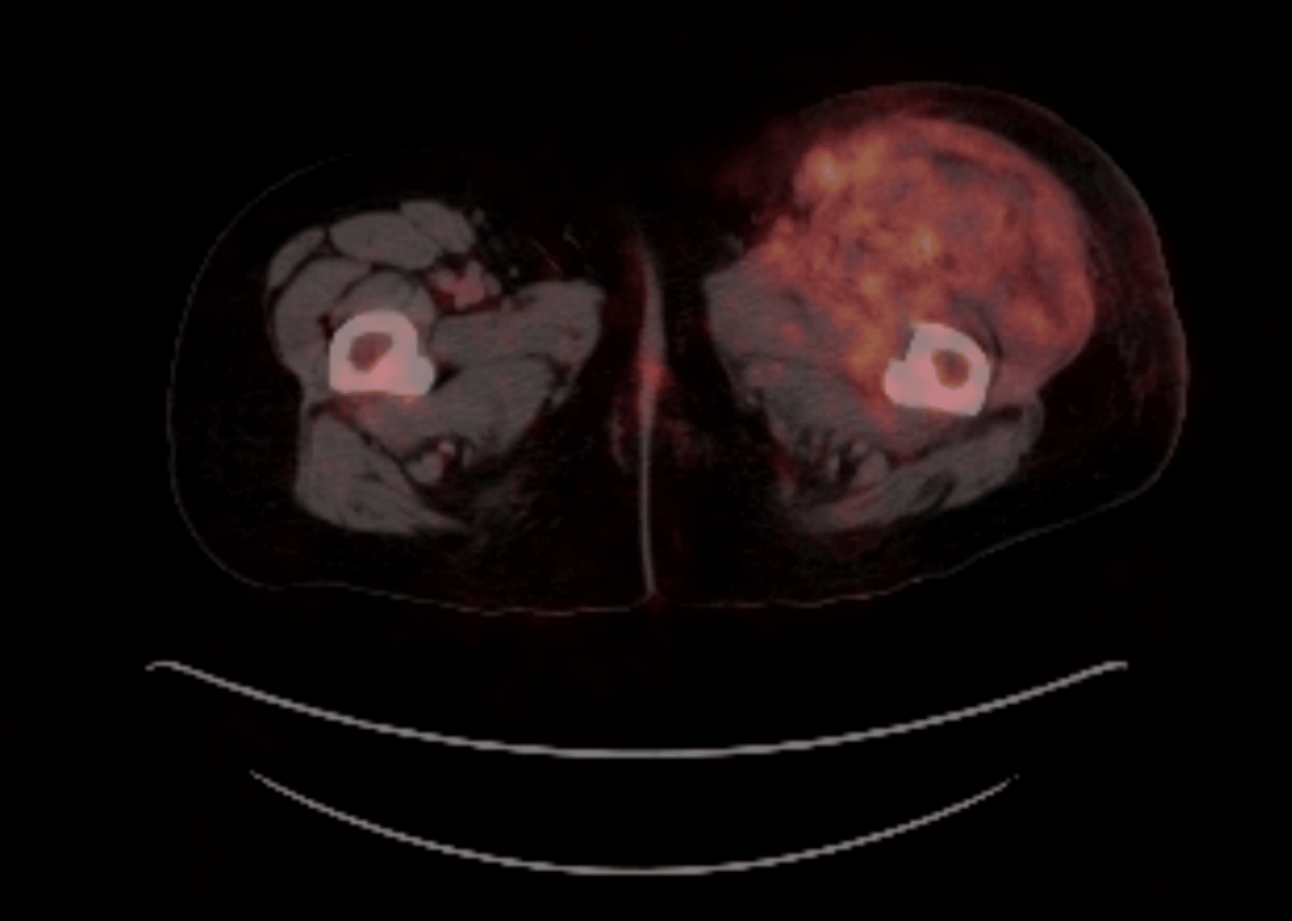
Axial FDG-PET/CT of the left thigh showing intense uptake in the quadriceps and adductor muscles, consistent with active inflammation. FDG, fluorodeoxyglucose; PET/CT, positron emission tomography/computed tomography.
